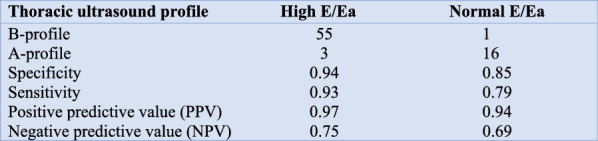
Table 1 Chest ultrasound profiles-based spectral tissue Doppler echocardiography E/Ea
